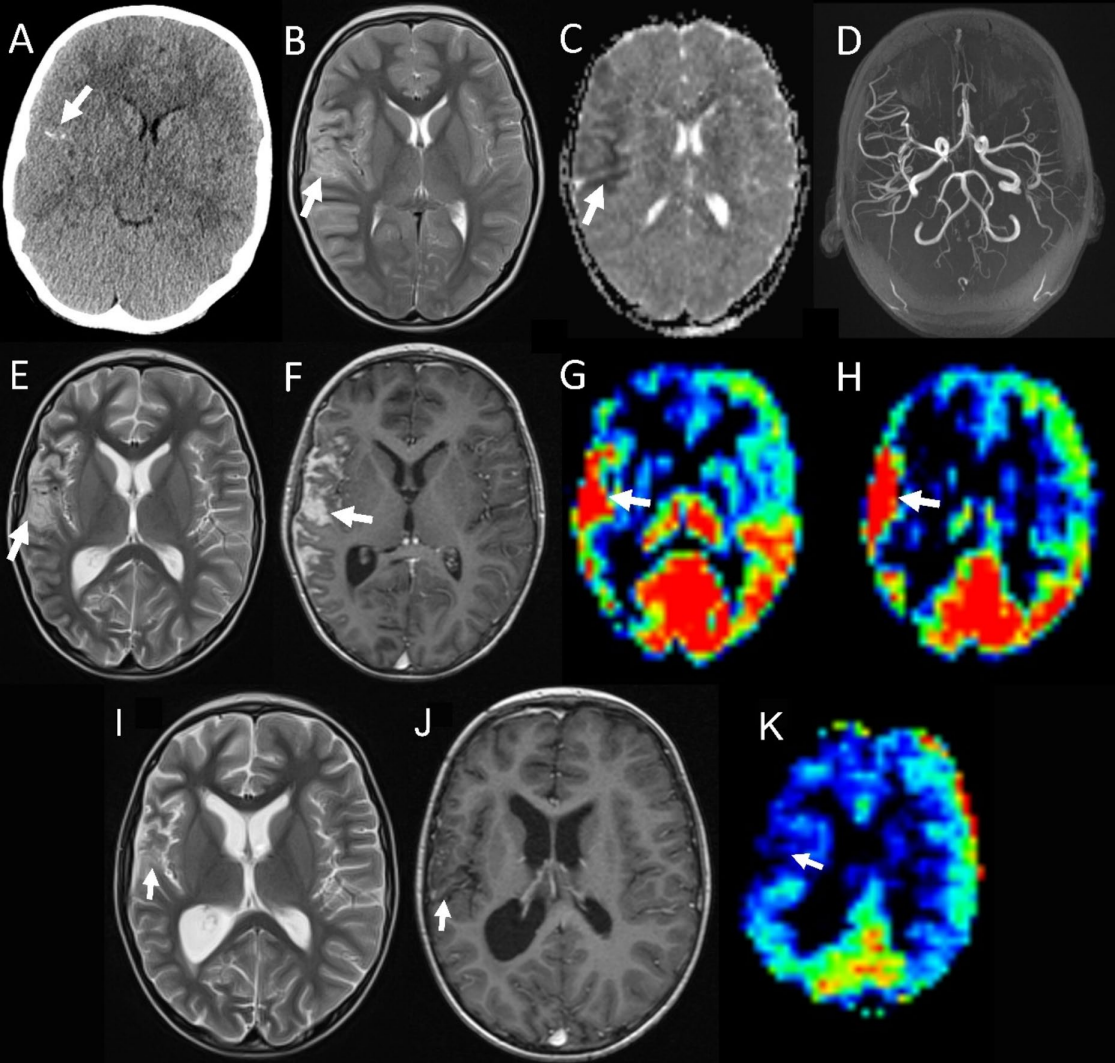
Presentation and primary treatment imaging. Non-contrast CT demonstrated what was initially thought to represent a right MCA thrombus occlusion (panel A arrow). MR imaging 1 day later demonstrated increased oedema on axial T2 sequences and associated diffusion restriction in an MCA distribution on the ADC map (panels B and C respectively, arrows). Yet, no major vessel occlusion was seen on the time-of-flight MRA (panel D). Panels E–H are taken from the re-presentation MRI scan some 5 weeks later. Axial T2 sequences showed worsening oedema (panel E, arrow). The T1 post-contrast image (panel F) showed significant leptomeningeal thickening and enhancement (white arrow) but no mass lesion. Concurrent ASL demonstrated significantly increased perfusion mapping to the leptomeningeal disease (panels G and H, arrows). By the end of therapy, the axial T2 (panel I) showed a reduction in peri-sylvian oedema (arrow) with a resolution to the previously seen contrast enhancement (panel J, arrow). This area also demonstrated post-treatment volume loss. The ASL sequence shows resolution to the previously seen increased perfusion (panel K, arrow, versus G and H; see Supplementary Information)
